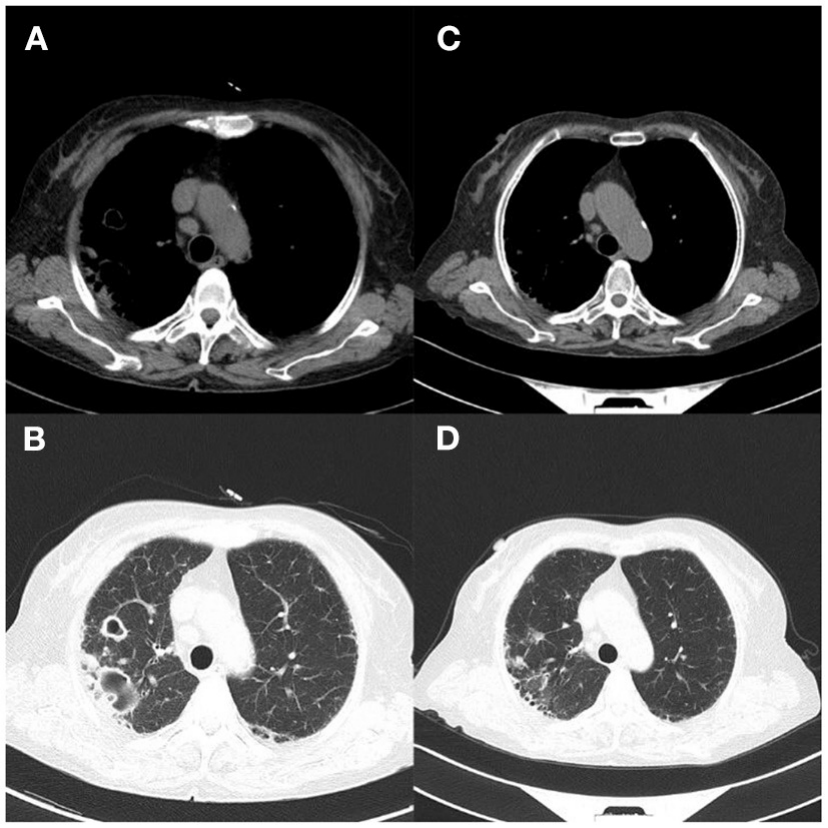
Case 2. (A,B) Lung CT on the day of admission showed interstitial inflammation in both lungs and cavity formation in the upper lobe of the right lung, localized emphysema of both lungs, mediastinal lymph node enlargement, and bilateral localized pleural thickening; (C,D) 1 month later, CT examination showed that the double pneumonia was slightly more advanced than before.
